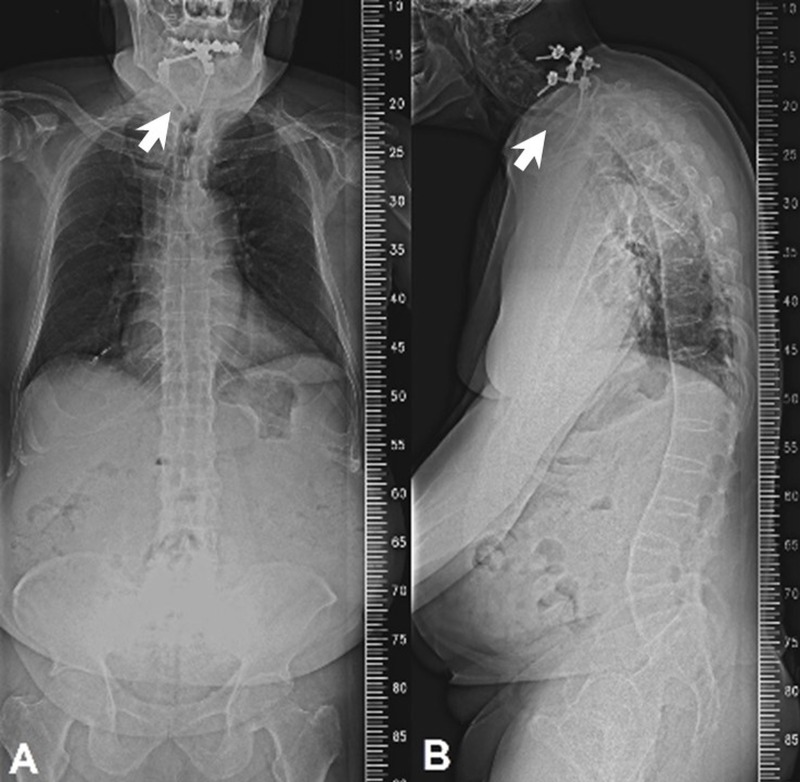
36-inch radiograph down to the pelvis A: Anterior-posterior view of a 36-inch radiograph down to the pelvis, demonstrates no visible cage in the chest or abdomen. B: Lateral view of a 36-inch radiograph down to the pelvis, demonstrates no visible cage in the chest or abdomen.
